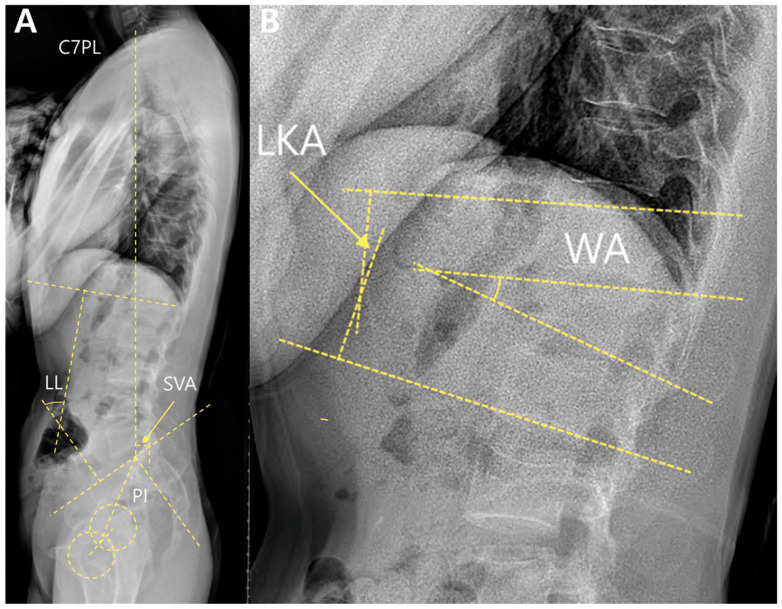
Measurements of spinal parameters. (A) Standing whole-spine lateral radiograph of a patient with an L1 burst fracture; (B) Magnified lateral view at the fracture level. The wedge angle (WA) was measured between the upper and lower endplates of the fractured vertebra, and the local kyphotic angle (LKA) was measured between the superior endplate of the vertebra above and the inferior endplate of the vertebra below. Abbreviations: C7PL, C7 plumb line; SVA, sagittal vertical axis; LL, lumbar lordosis; PI, pelvic incidence; WA, wedge angle; LKA, local kyphotic angle.
